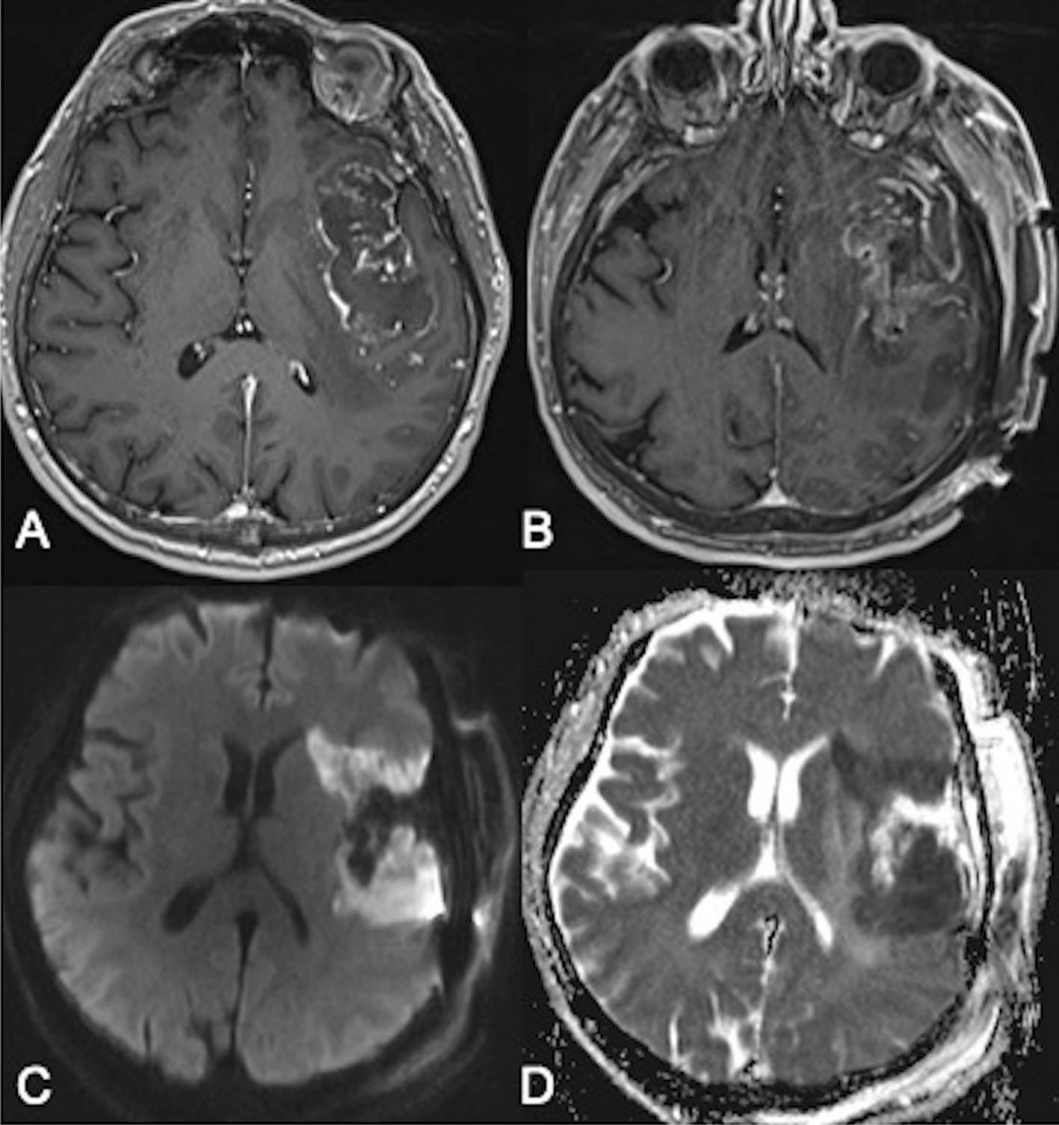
T1-weighted MRI with contrast of a 64 year-old male with an insular glioblastoma before (A) and after (B) surgery. Post-operative diffusion-weighted imaging (C) and apparent diffusion coefficient (D), showing a large wedge-shaped surgery-related infarct.
